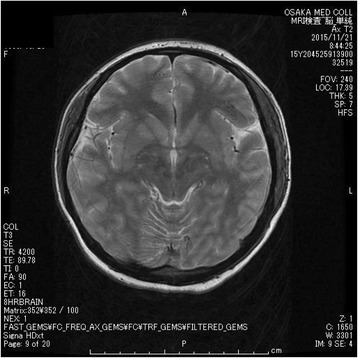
Brain MRI image with no remarkable findings
